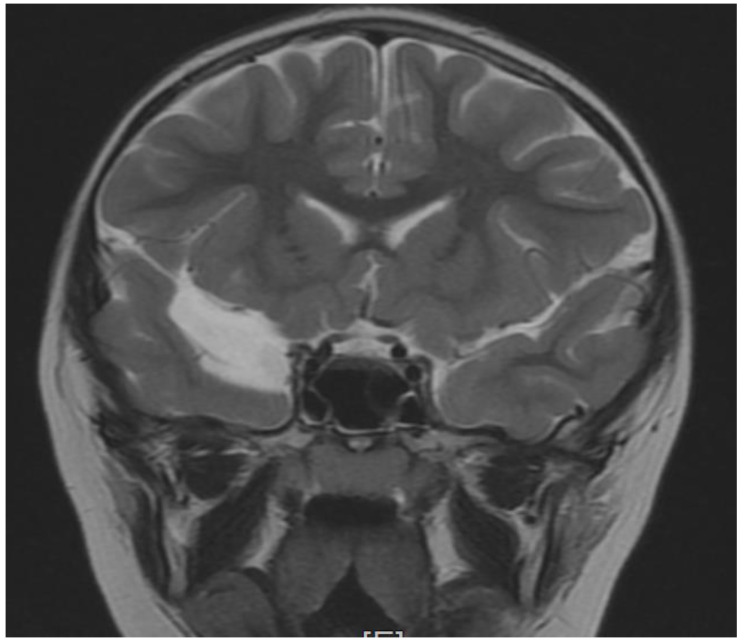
Coronal (T2-weighted sequence)—cyst—at the front of the temporal lobe.
